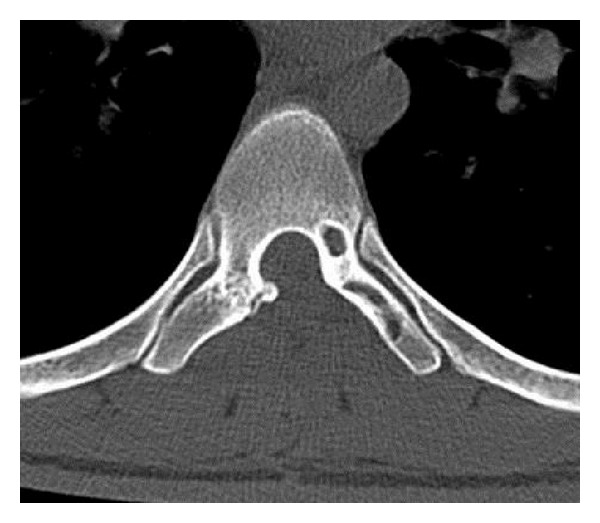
Postoperative 2-year follow-up axial CT scan: the eroded bone areas are in a healing process.
